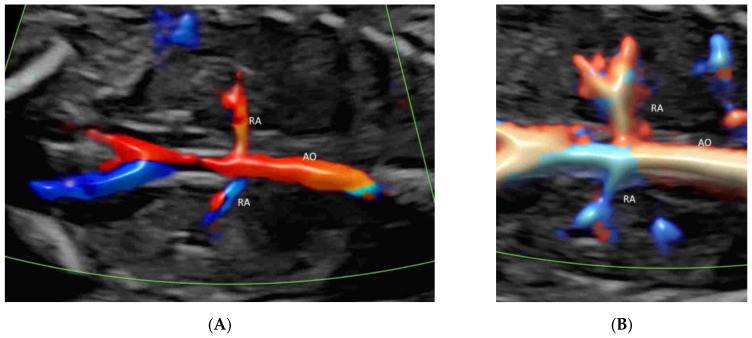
Color Doppler (A) and high-definition (B) flow imaging in a coronal plane through the back of a normal fetus at 20 weeks’ gestation showing both renal arteries (RA) arising from the aorta (AO).
